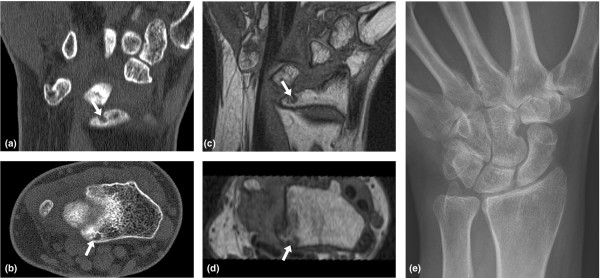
Erosions in the wrist of a rheumatoid arthritis patient. Wrist of a rheumatoid arthritis patient visualised by (a, b) computed tomography and (c, d) T1-weighted magnetic resonance imaging in the (a, c) coronal and (b, d) axial planes. A bone erosion at the distal radius is seen on both computed tomography and magnetic resonance images in two planes (white arrows), but not on the corresponding radiograph (e). The erosion was assigned an OMERACT erosion score of 1 on both computed tomography and magnetic resonance imaging.
